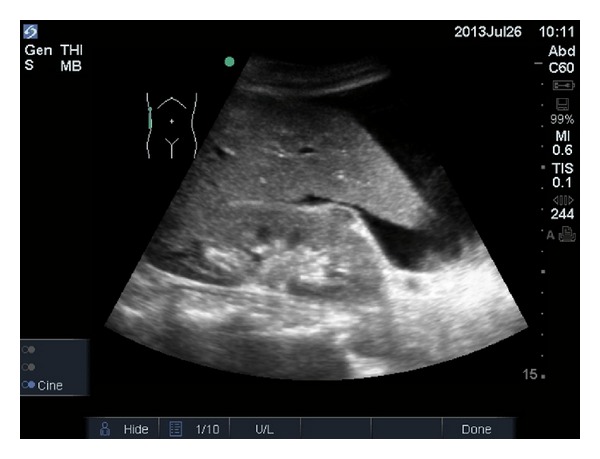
Free intra-abdominal fluid on ultrasound.
